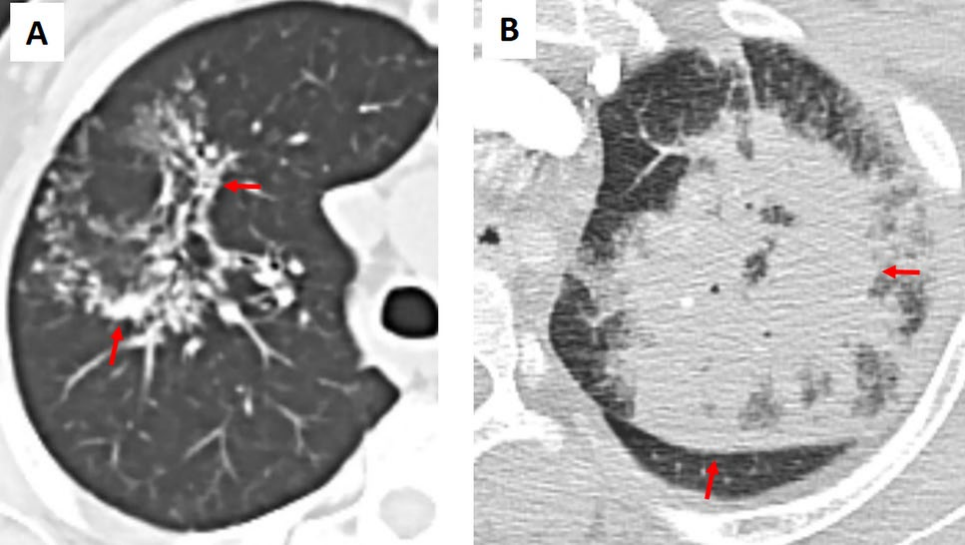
Reverse halo (atoll) sign (arrows) in two different patients with sarcoidosis. A Axial chest CT image shows multiple tiny coalescing nodules in the right upper lobe forming the atoll sign. B Axial chest CT image of a patient with alveolar sarcoidosis demonstrates left upper lobe consolidation with central ground-glass opacities consistent with the atoll sign
